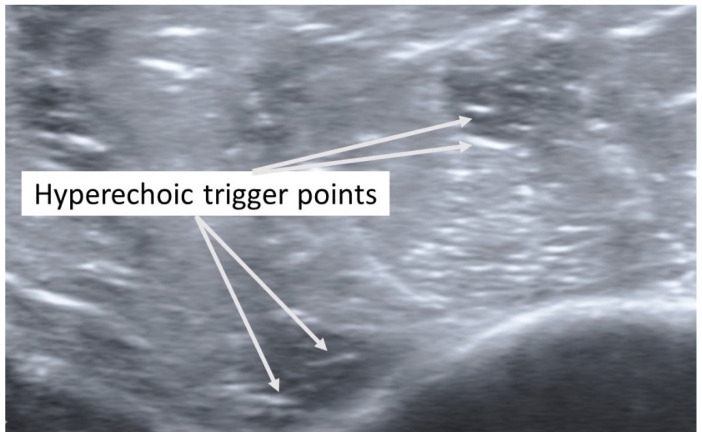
Detailed ultrasound imaging assessment of the right deltoid muscle showing a collection of small hyperechoic (hypoperfused) “TrP speckles” within each of the contracture knots.
